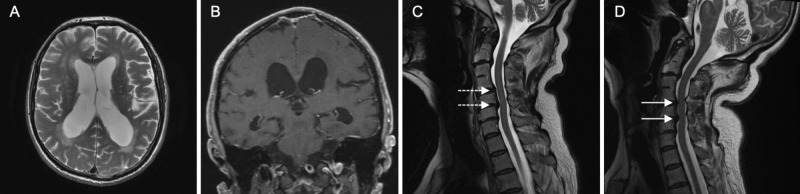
Patient’s Brain and Cervical Spine MRI (A) Axial T2-weighted brain MRI demonstrating mild ventriculomegaly with Evan’s index 0.32. (B) Coronal T1-weighted contrast-enhanced brain MRI demonstrating enlargement of the temporal horns, tight-high convexity and medial surface subarachnoid spaces, enlarged left Sylvian fissure, and a callosal angle measuring 73.9°. (C) Sagittal T2-weighted cervical spine MRI; dotted arrows indicate mild stenosis at C4-C5 and moderate stenosis at C5-C6 with the patient’s head in the neutral position. (D) Sagittal T2-weighted cervical spine MRI; solid arrow depicts severe stenosis at C4-C5 and C5-C6 in extension.
